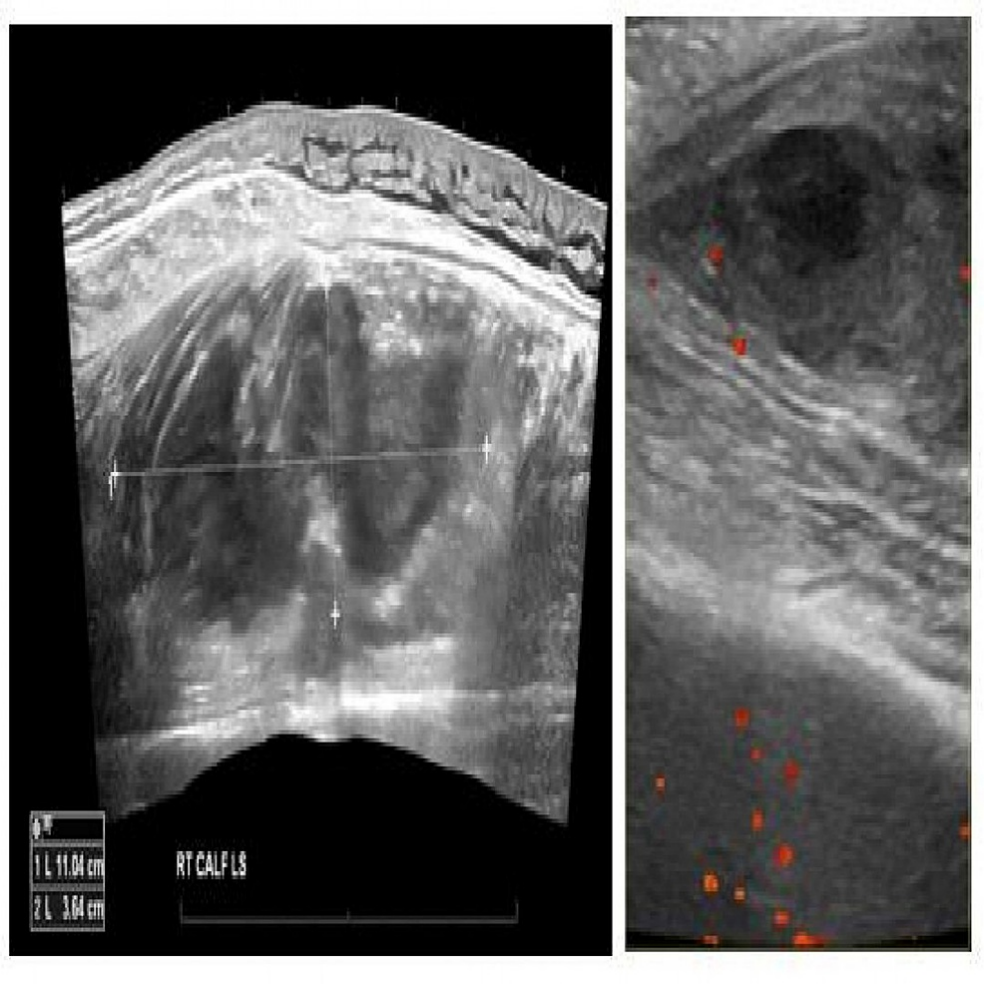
Ultrasonography scan. Left: The right calf demonstrates hematoma, 57 x 45 x 110 mm in length. Right: A large defined intramuscular hypoechoic lesion, 30 x 30 x 85 mm in length, appears to lie within the biceps muscle belly. The appearance is suggestive of hematoma. No thrombus was seen.
